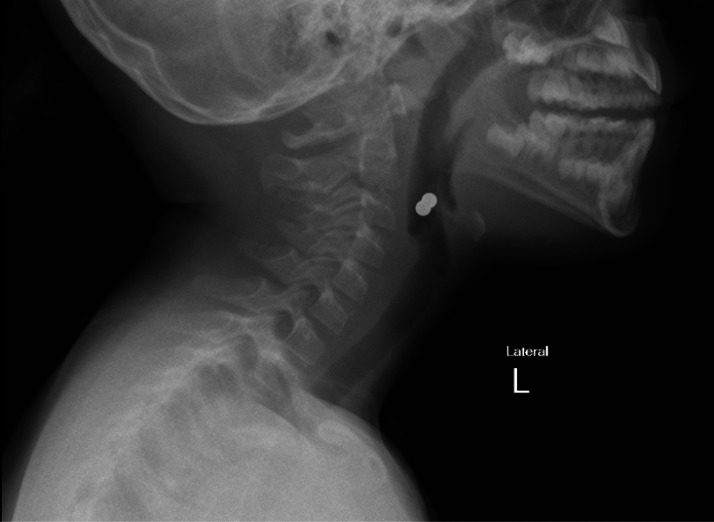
X-ray neck (lateral view), rare earth magnets clumped with pharyngoepiglottic fold
